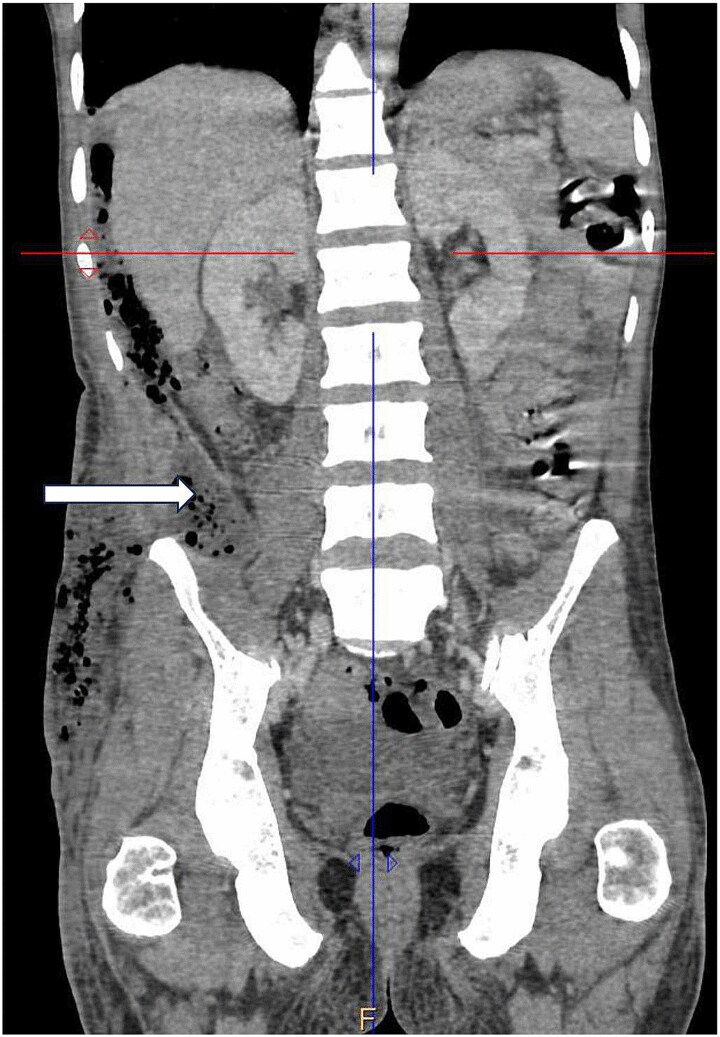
Preoperative contrast-enhanced CT abdomen and pelvic coronal image showing extensive infected collections extending from the retrocecal region into the right paracolic gutter (arrow) and retroperitoneal spaces, with superior extension toward the perihepatic region.
